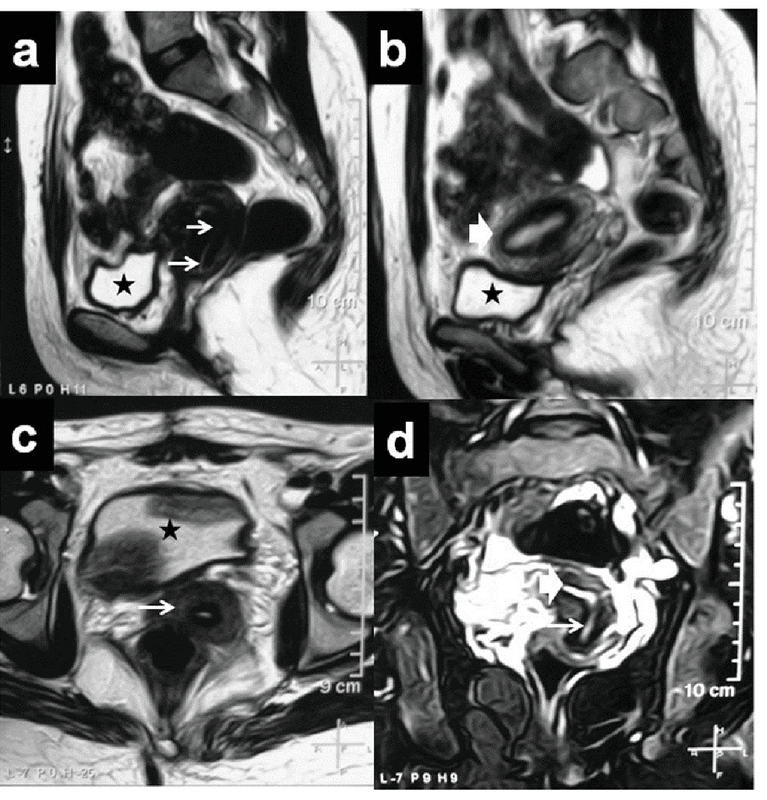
A six-month follow-up MRI.
